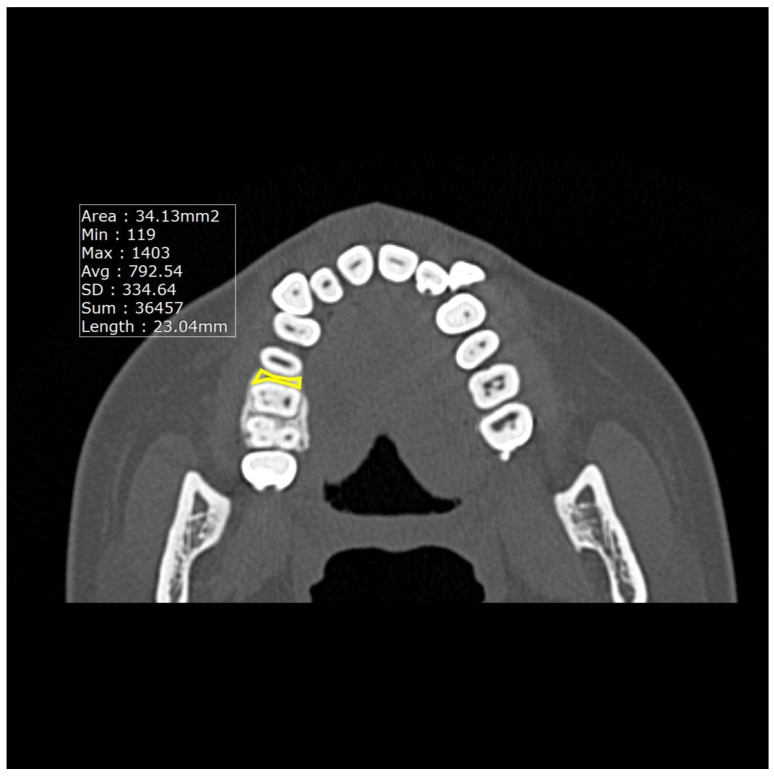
Image showing the measurement of the average CT values in the maxilla.
